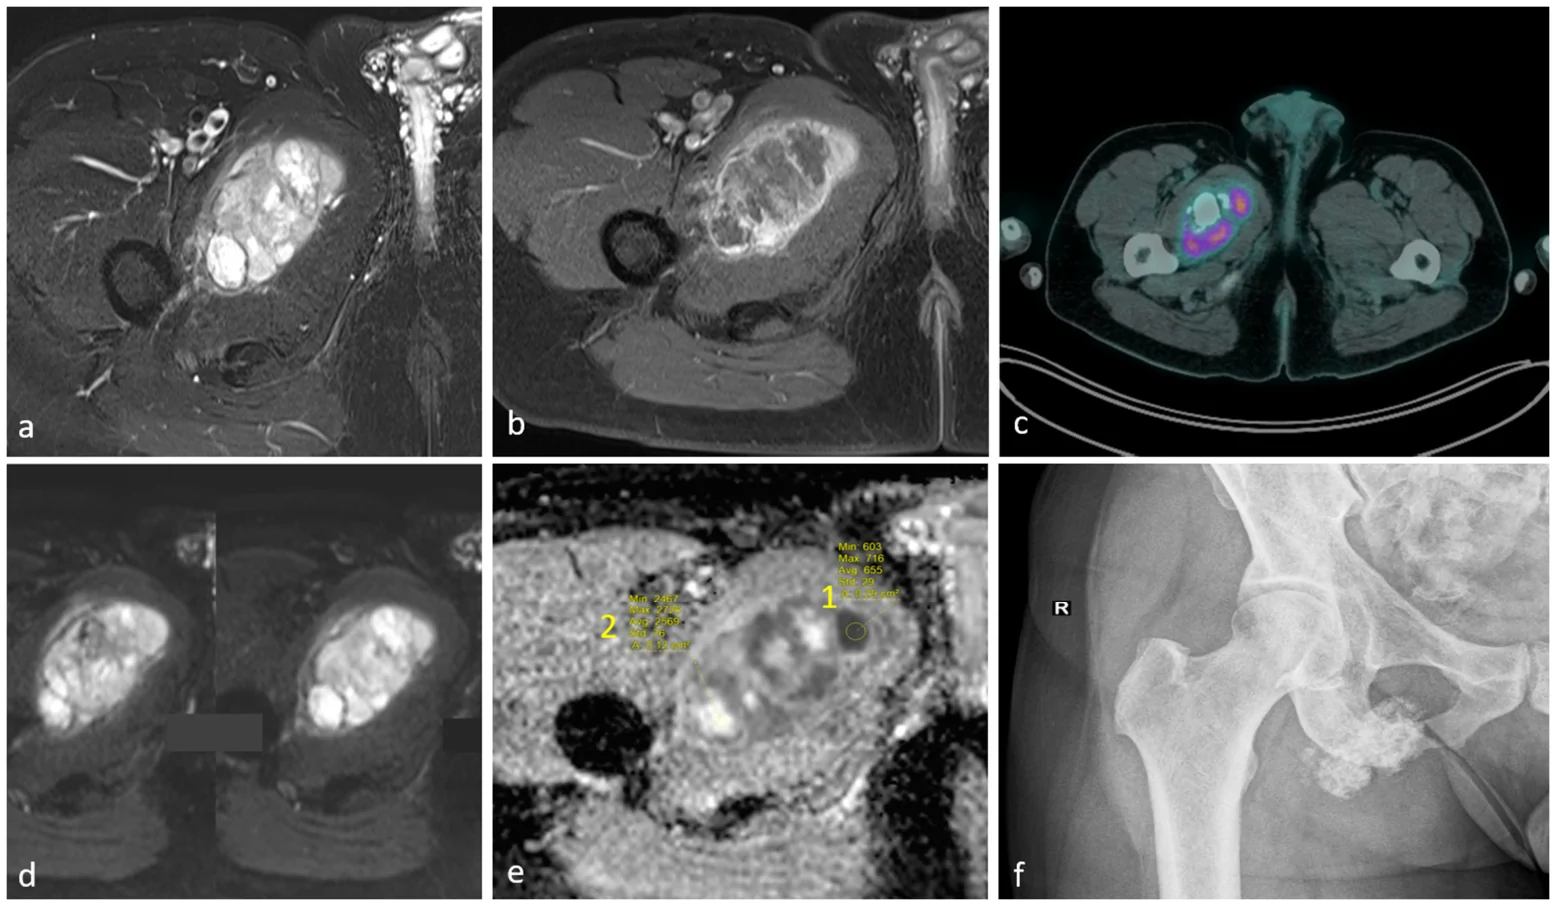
Axial MRI, PET-CT, and radiograph of a biopsy-proven extraskeletal mesenchymal chondrosarcoma. Fat-suppressed T2-weighted imaging (a) demonstrates a heterogeneous mass with mixed signal intensity. Post-contrast T1-weighted imaging (b) reveals peripheral enhancement with a central peripheral and septal chondroid pattern of enhancement. DWI (d) shows heterogeneous bright signal, with the ADC map (e) identifying an eccentric focus of true restricted diffusion corresponding to the round cell sarcomatous component (1) and a separate focus of T2 shine-through representing the chondroid component (2). FDG PET-CT (c) confirms peripheral metabolic activity with central calcification, and the frontal radiograph of the right hip (f) demonstrates characteristic ring-and-arc calcifications. This case highlights the role of DWI in characterising tumour heterogeneity—the sarcomatous component exhibits restricted diffusion and avid enhancement, whilst the chondroid component is responsible for T2 shine-through and the characteristic calcification pattern.
